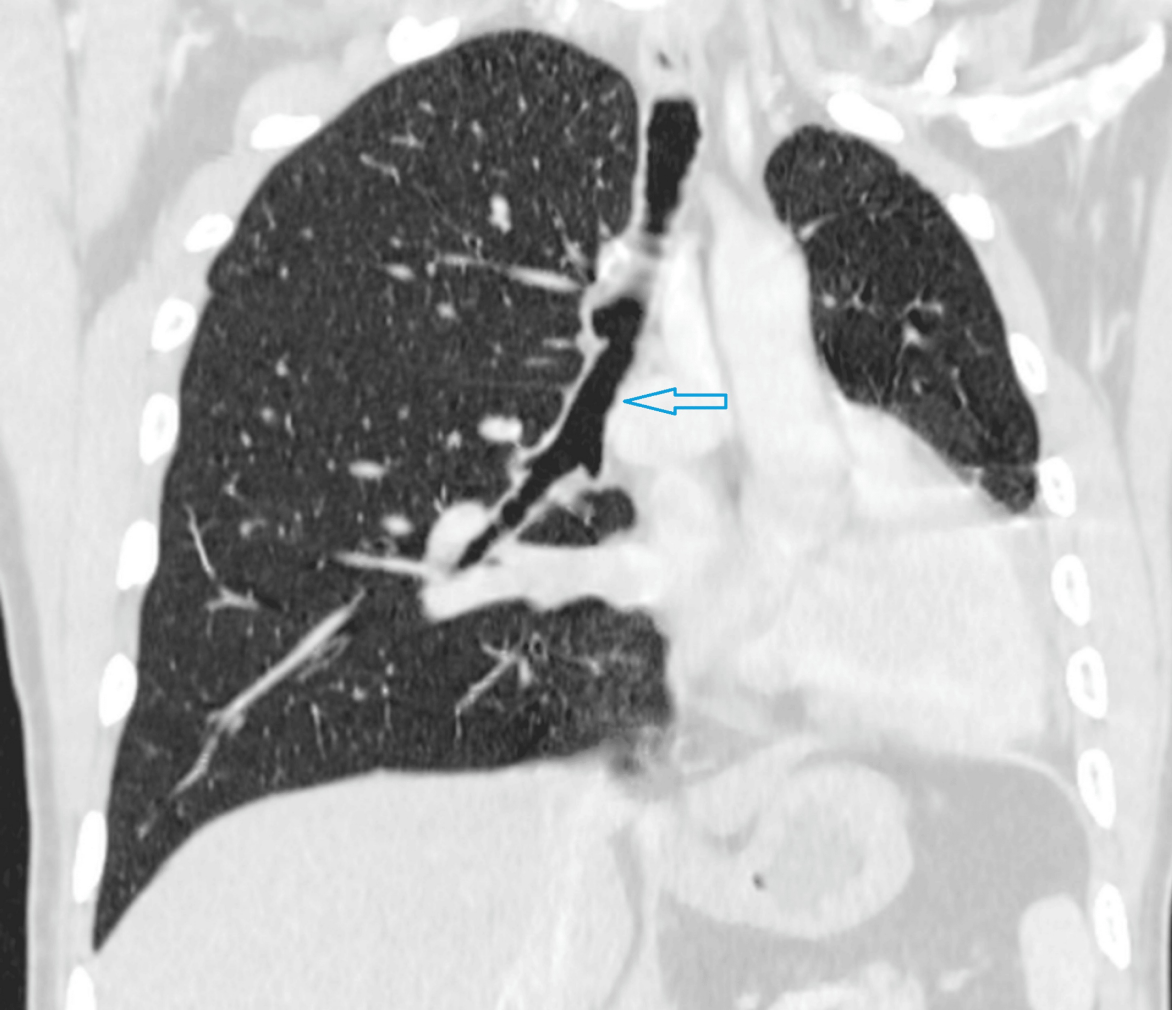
CT scan of the chest in the lung window and coronal reconstruction shows absent left main bronchus CT: computed tomography
